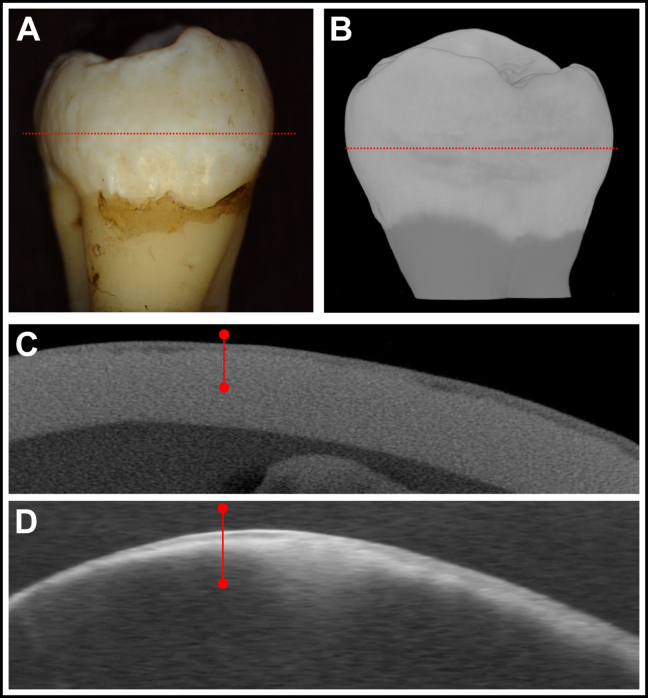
Color image (A) and microcomputed tomographic (B) surface rendering of the buccal surface of a tooth with mild fluorosis showing the position at which the microcomputed tomographic (C) and processed optical coherence tomographic (D) cross-sectional images shown were extracted from the position of the dotted red line. The solid red lines show the position that was chosen for analysis.
